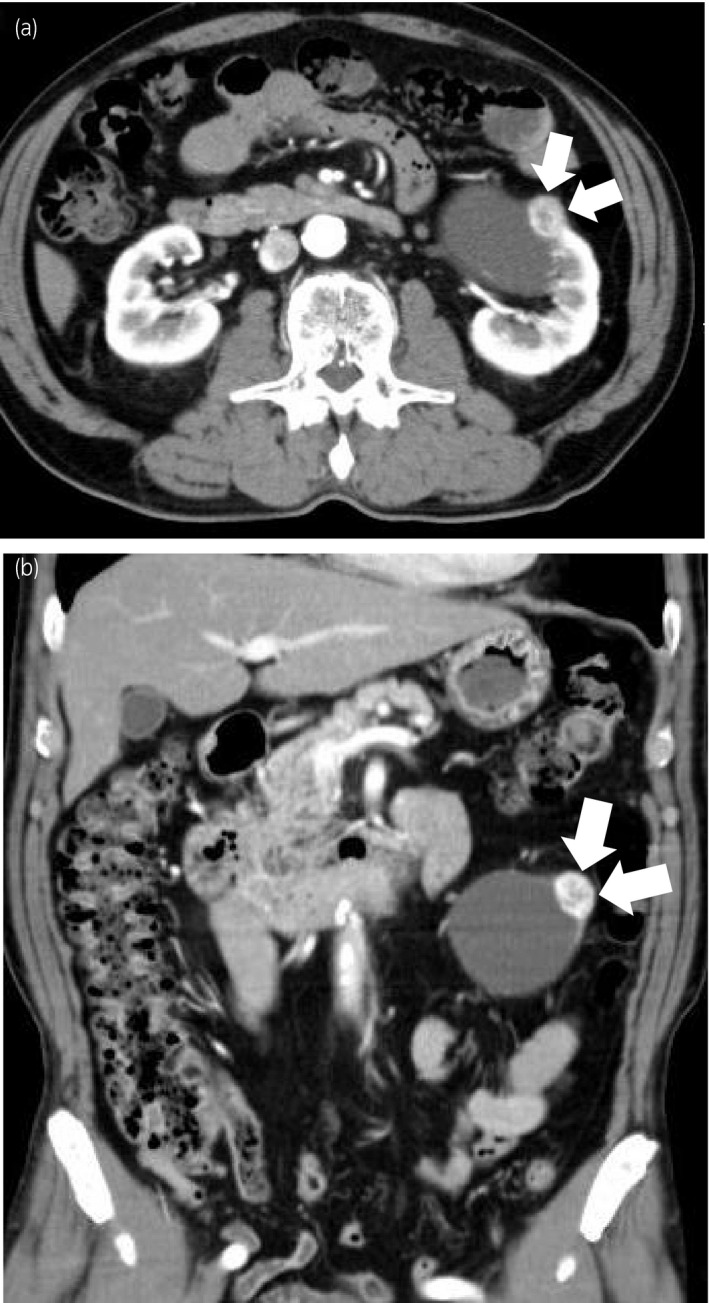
Contrast‐enhanced CT showed an enhanced tumor adjacent to a renal cyst in the left kidney. Arrows: tumor. (a) Axial section. (b) Coronal section.
